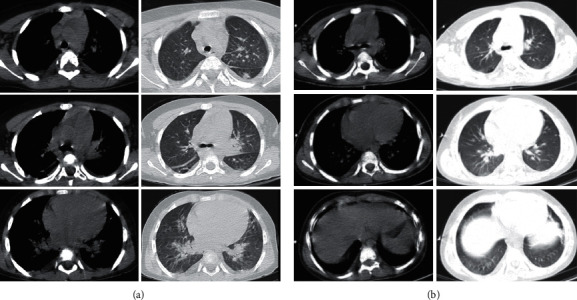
(a) The computed tomography (CT) scan depicts increasing vascular marking, interlobular septal thickening, ground-glass opacities, and mild bilateral pleural effusion. (b) A second computed tomography of the patient is shown here. There is no evidence of pleural effusion or pleural thickening, and the ground-glass opacities got almost clear.
